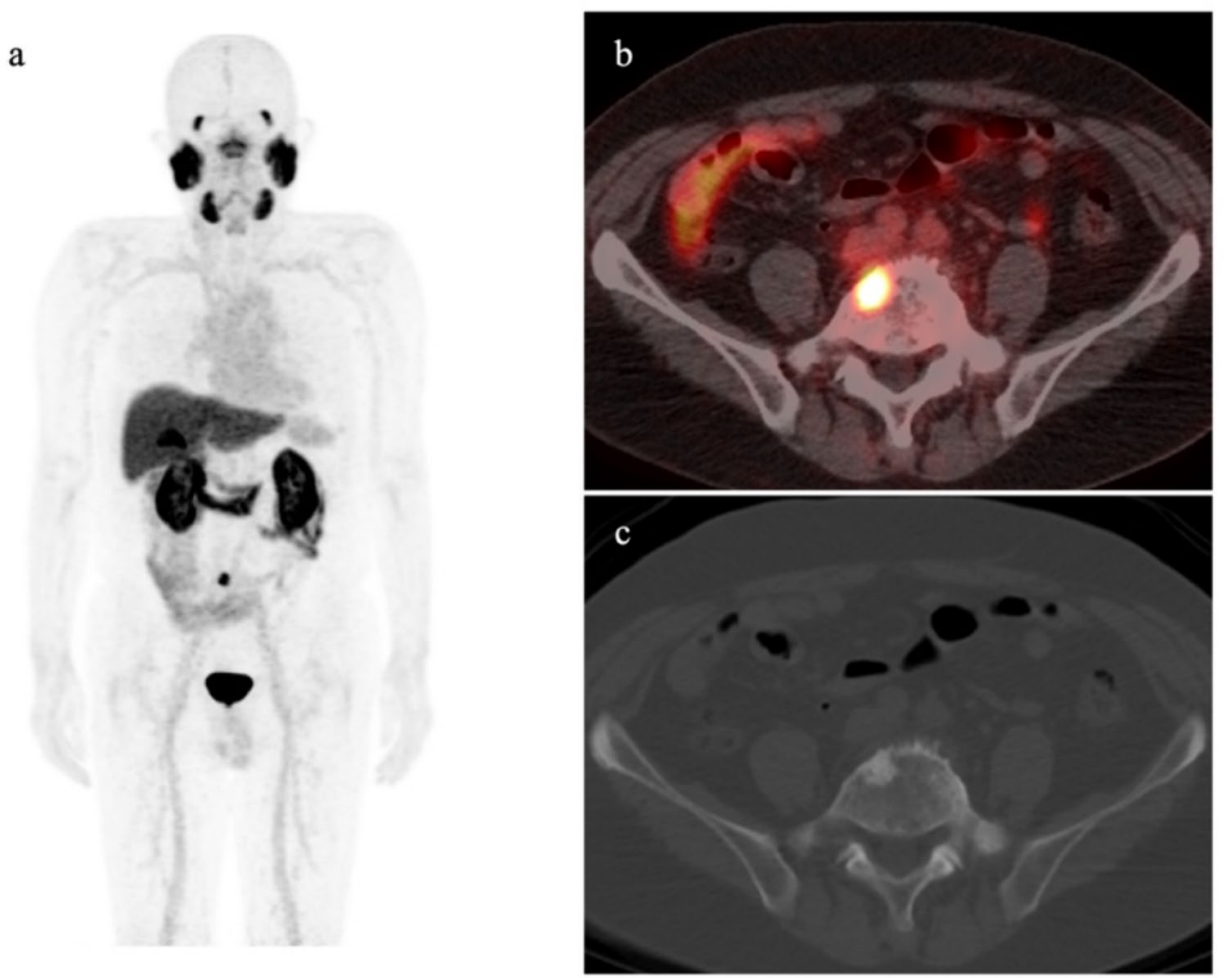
A 74-year-old man who had previously undergone total prostatectomy (pT2cN0, Gleason score 3 + 4) demonstrated biochemical recurrence. PSA level elevated to 1.44 ng/ml. PET/CT with ^18^F-FSU-880 showed focal uptake in the lumbar spine (SUVmax: 6.9), suggesting bone metastasis. As the metastasis was solitary, metastasis-directed therapy to the bone metastasis was performed, resulting in a decrease in PSA to 0.05 ng/ml. a Maximum intensity projection image. b Transaxial PET/CT fusion image. c Transaxial CT
